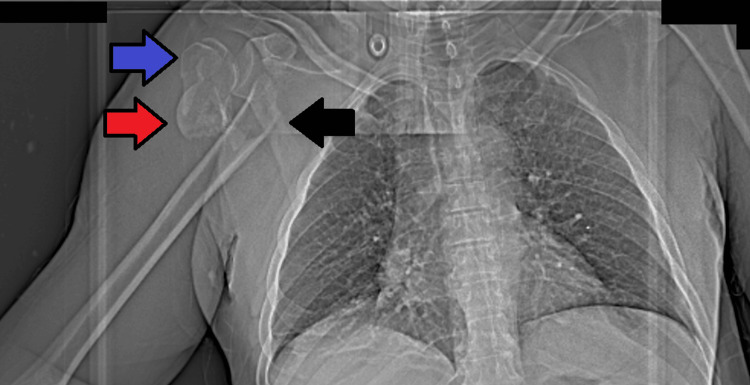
Anteroposterior scout view of chest CT showing a complex fracture of the right proximal humerus. The blue and red arrows indicate the splitted humeral head and the black arrow highlights the displaced proximal humeral shaft in the axilla.
